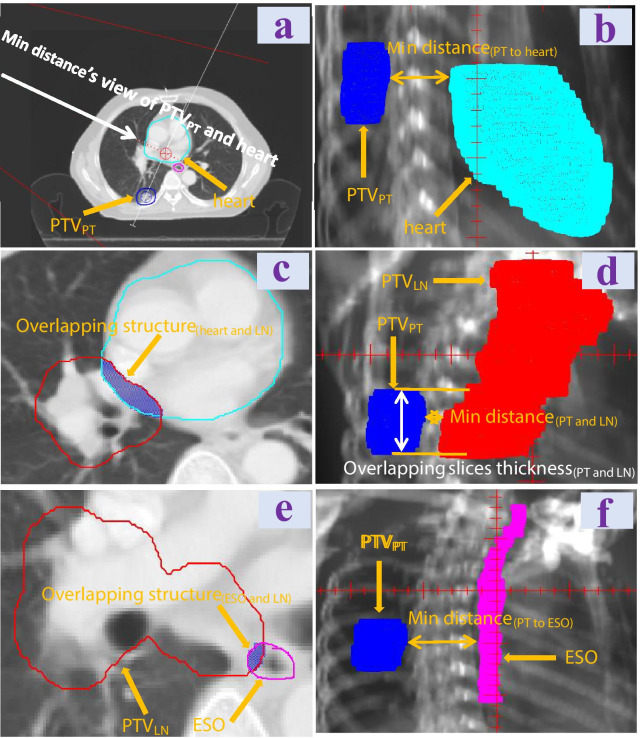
Anatomical geometry features shown on CT images and min distance view’s DRR images. a Min distance’s view of PTV_PT_ and heart (the view direction is vertical to the centers’ connection of PTV_PT_ and heart on CT slice); b Min distance_(PT to heart)_ shown on corresponding min distance view’s DRR image; c Volume ratio of overlapping structure_(heart and LN)_ to heart or PTV_LN_; d Min distance_(PT to LN)_ and overlapping slices thickness_(PT and LN)_ shown on corresponding min distance view’s DRR image; e Volume ratio of overlapping structure_(ESO and LN)_ to ESO; and f Min distance_(PT to ESO)_ shown on corresponding min distance view’s DRR image
